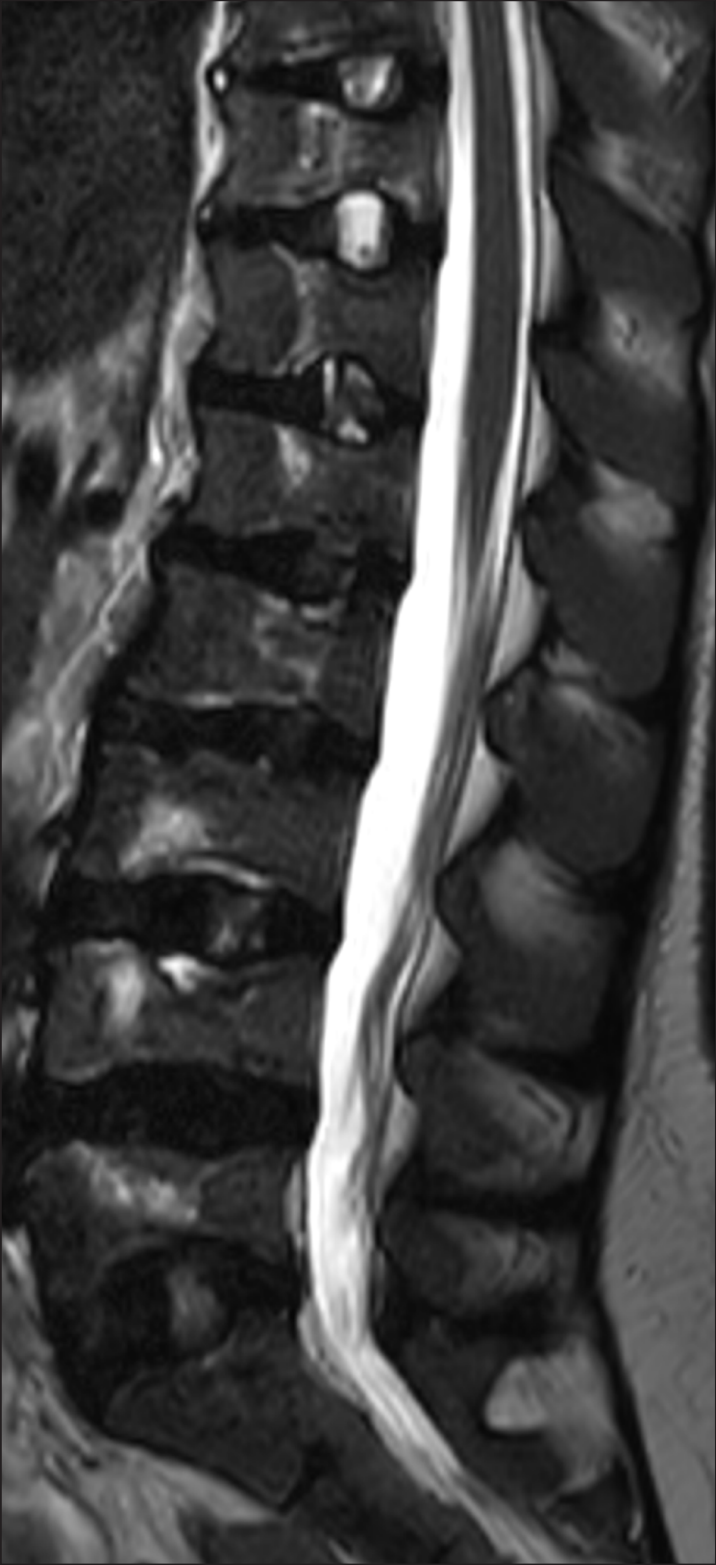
MRI also showed multiple T2 hyperintense central lesions in several vertebral bodies, which represent bone marrow edema due to bone infarctions (Figures 1 and 2).
